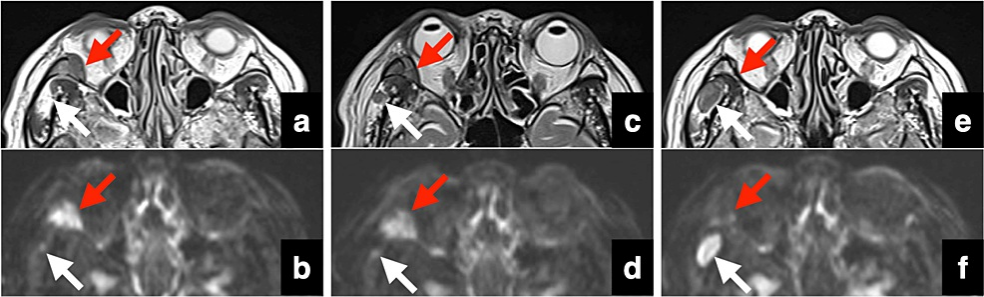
Time course of the right orbital lesion (red arrows) and temporal fossa (white arrow) before and after CKR on MRI T2WI and DWI pre-CKR: (a) T2WI, (b) DWI. Day 5 after starting CKR: (c) T2WI, (d) DWI. Day 44 after starting CKR: (e) T2WI, (f) DWI CKR: CyberKnife radiotherapy, MRI: magnetic resonance imaging, T2WI: T2-weighted image, DWI: diffusion-weighted image
